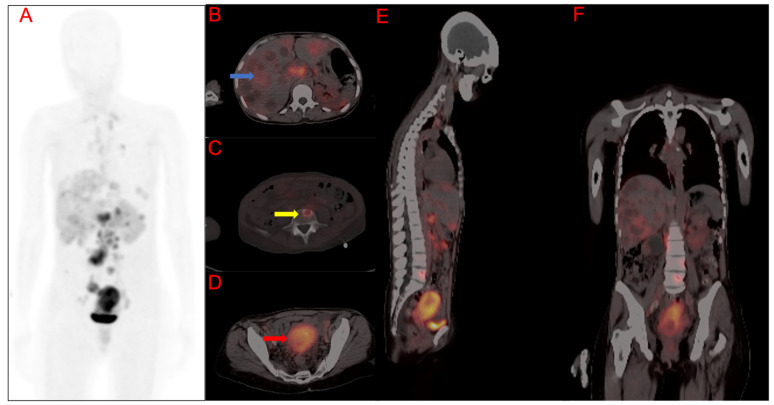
A 50-year-old female with poorly differentiated squamous cell carcinoma of the cervix. [^68^Ga]Ga-FAPI-46 PET/CT for staging demonstrated maximum intensity projected image (MIP) (A), fused PET/CT transaxial slice through the liver (B), fused PET/CT transaxial slice through the 4th lumbar vertebra (C), fused PET/CT transaxial slice through the pelvis (D), fused PET/CT whole-body sagittal (E), (blue arrow) and skeletal metastases (yellow arrow) and intense primary disease (red arrow) with uterine uptake, fused PET/CT whole-body coronal images demonstrate liver metastases (F). Primary cervix lesion SUVmax = 22.47, FAPI-TV = 115.54 cm^3^.
